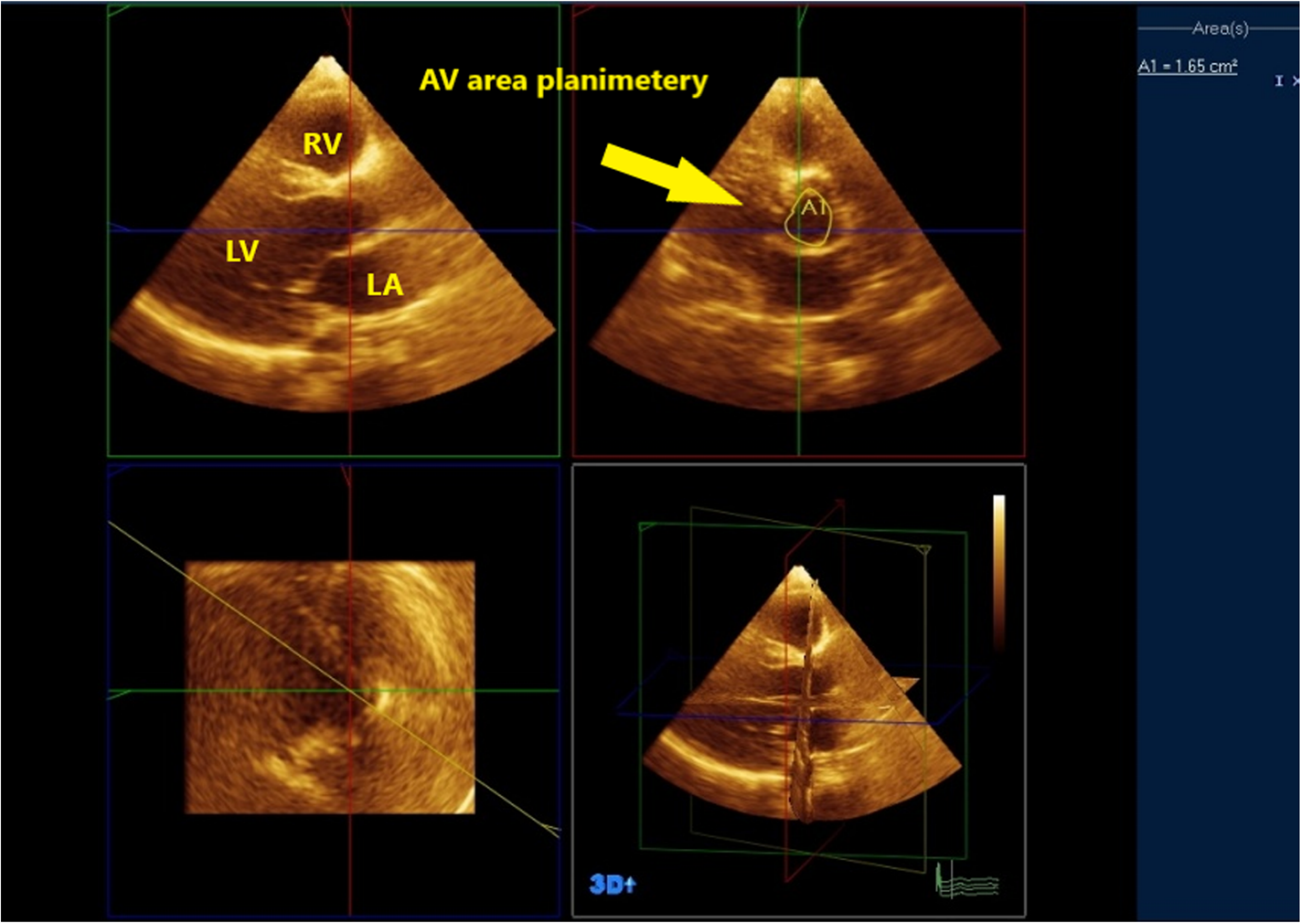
AVA was measured by direct planimetery in parasternal short axis view using MPR mode. In patient no. 1
